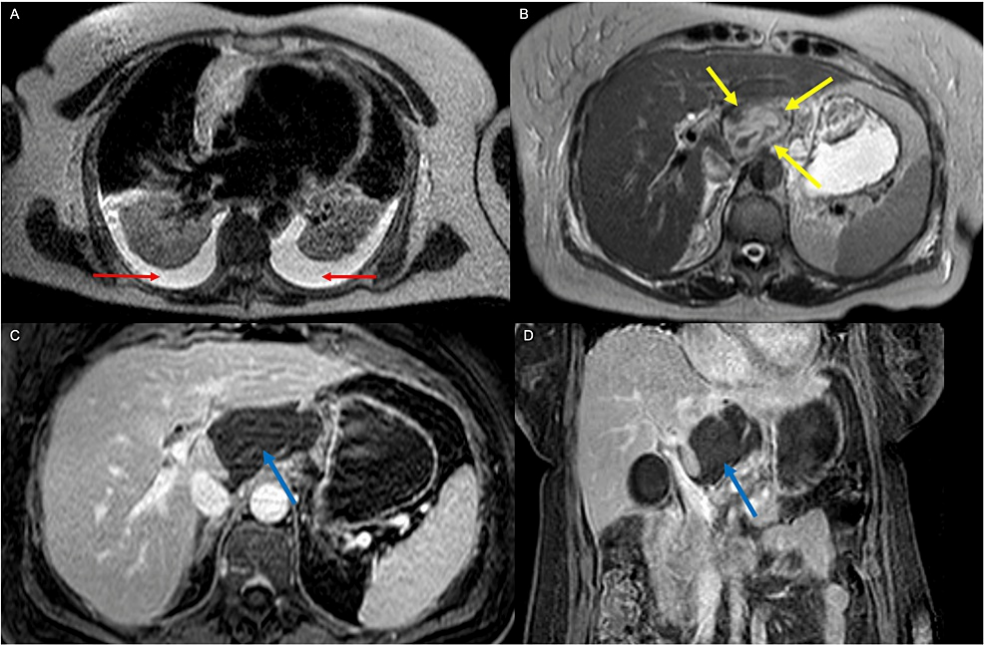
Axial MRI of the abdomen. T2-weighted images (A, B) demonstrate bilateral pleural effusions (red arrows in A) as well as a mixed-density heterogeneous mass arising from the caudate lobe of the liver (yellow arrows in B). Axial (C) and coronal (D) contrast-enhanced images demonstrated curvilinear enhancement of the caudate lobe mass with internal signal suggestive of proteinaceous or hemorrhagic components (blue arrows in C and D).
